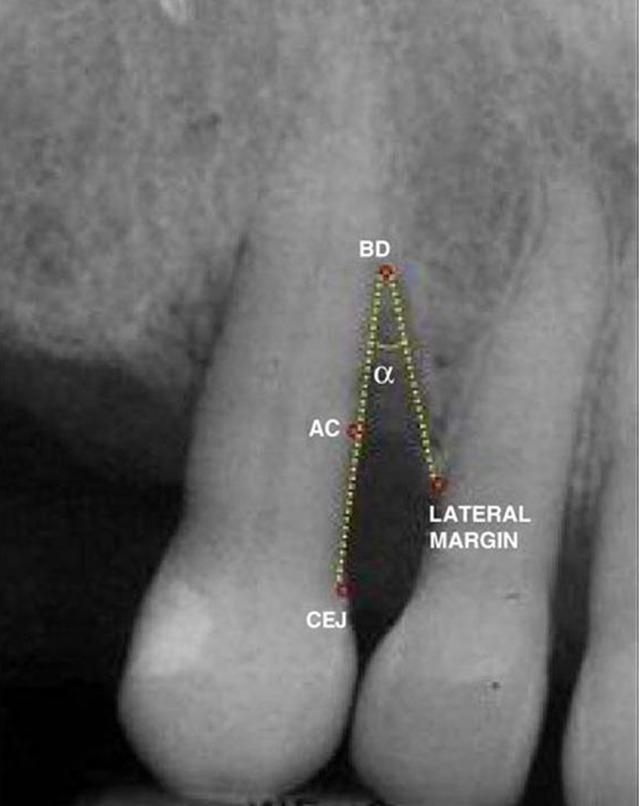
Radiographic measurements
